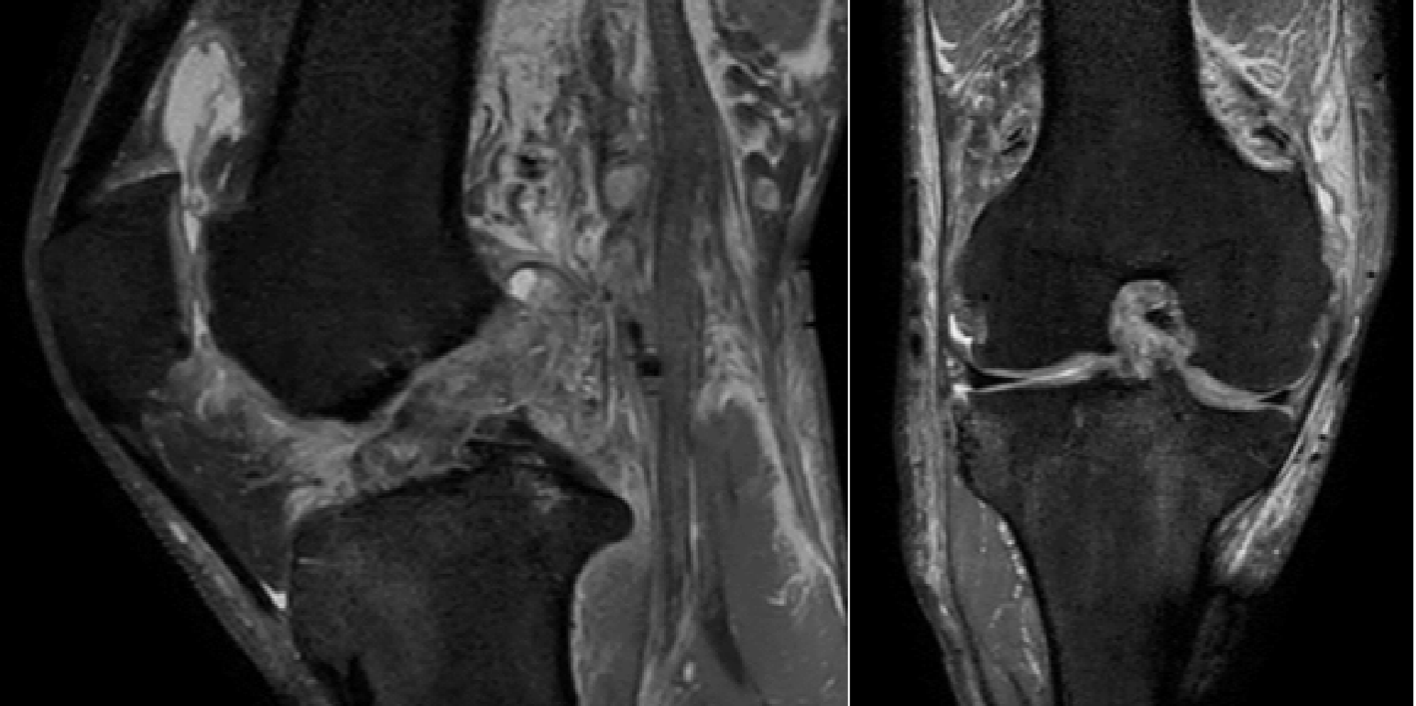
MRI image showing the ruptured ACL*.*
